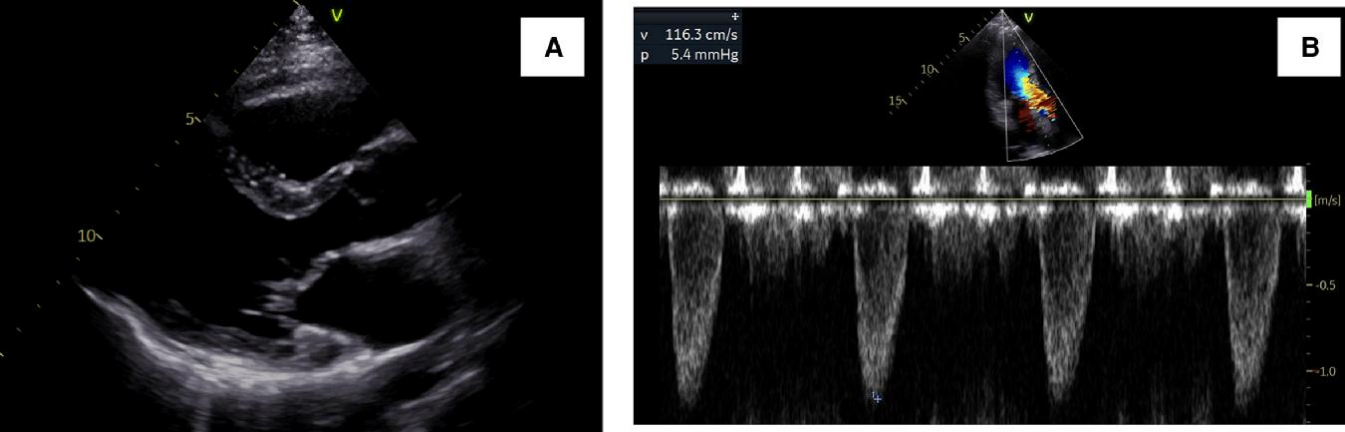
Postoperative transthoracic echocardiogram findings. (*A*) The high-echoic linear structure had vanished in long axis view of left ventricle. (*B*) Pulse-wave Doppler image showing blood flow in the left ventricular outflow tract. The maximum flow velocity was 1.16 m/s.
